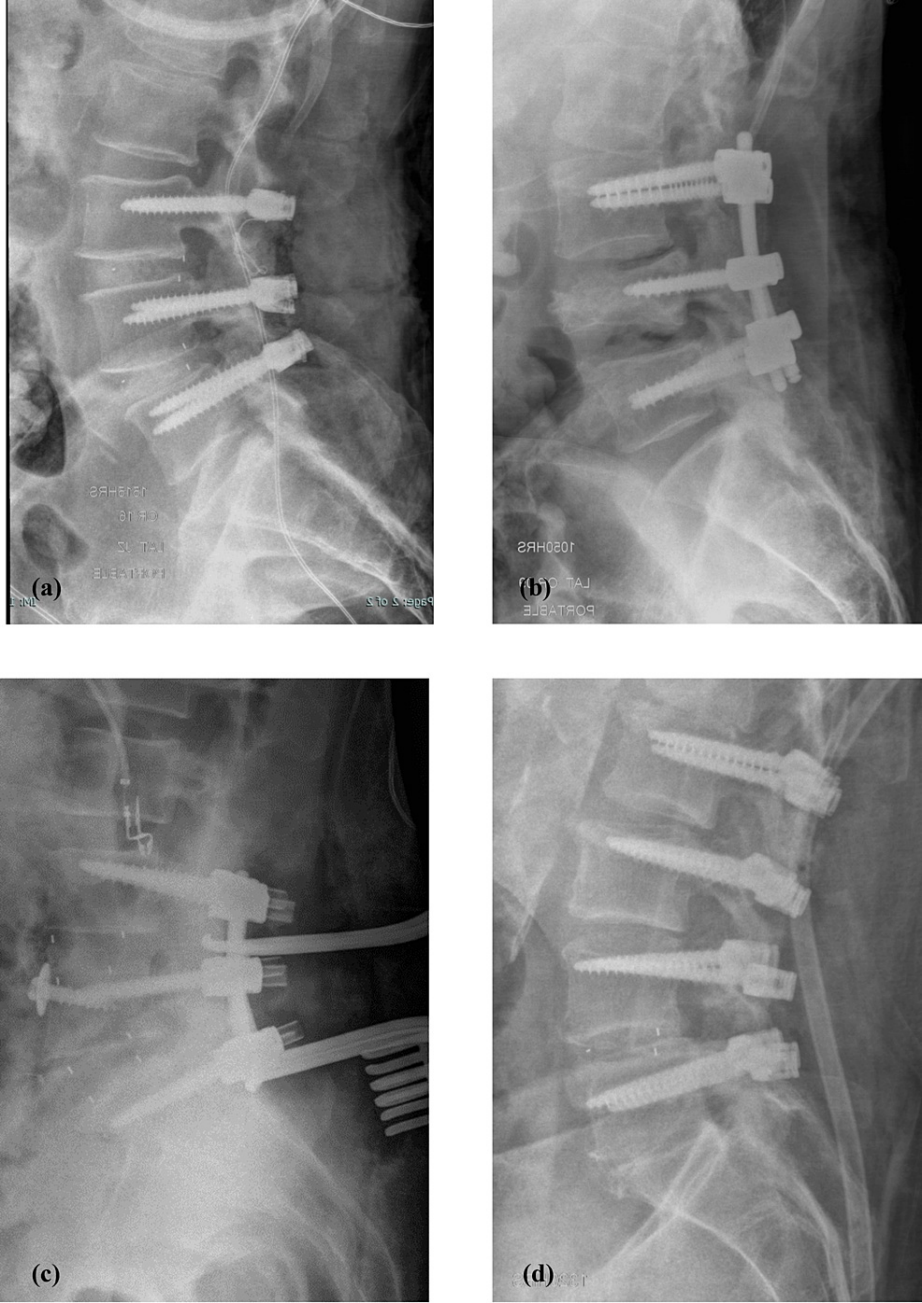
Lateral (LAT) radiographs - examples of (a) true positive (subject 1), (b) false negative (subject 4), (c) false positive (subject 113), and (d) true negative (subject 2)
